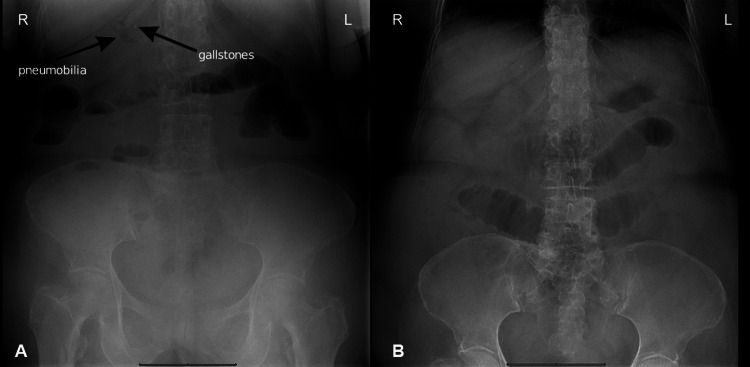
Plain abdominal radiograph: (A) standing and (B) sitting
